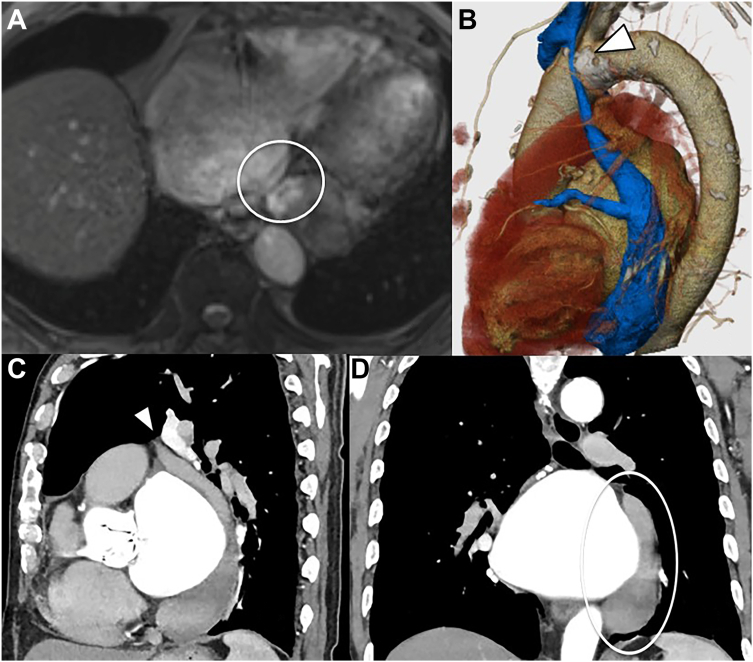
(A) Cardiac magnetic resonance image shows membranous closure of the coronary sinus ostium with no direct coronary sinus–right atrium communication (circle). Computed tomography (B) 3-dimensional, (C) sagittal, and (D) coronal views show long-segment stenosis at the persistent left superior vena cava–left brachiocephalic vein junction (B, C; arrowheads), with a markedly dilated great cardiac vein (D; oval) and near-absence of venous opacification (C, D).
